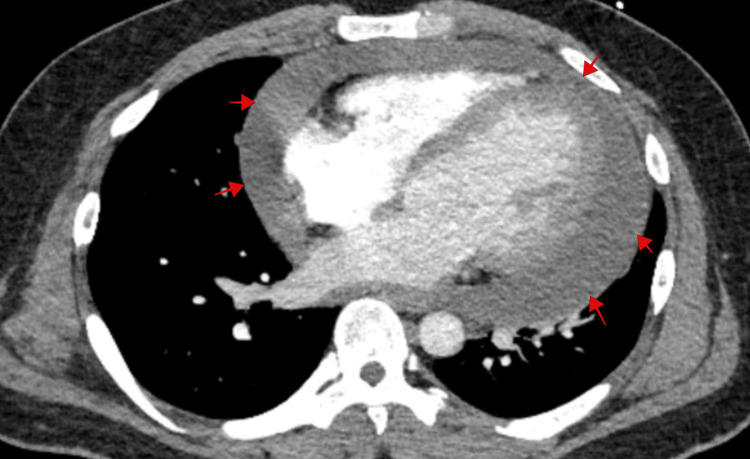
Computed tomography angiogram of the chest showing large pericardial effusion (red arrows) with borderline pericardial thickening and the presence of mild concavity of the left atrium
